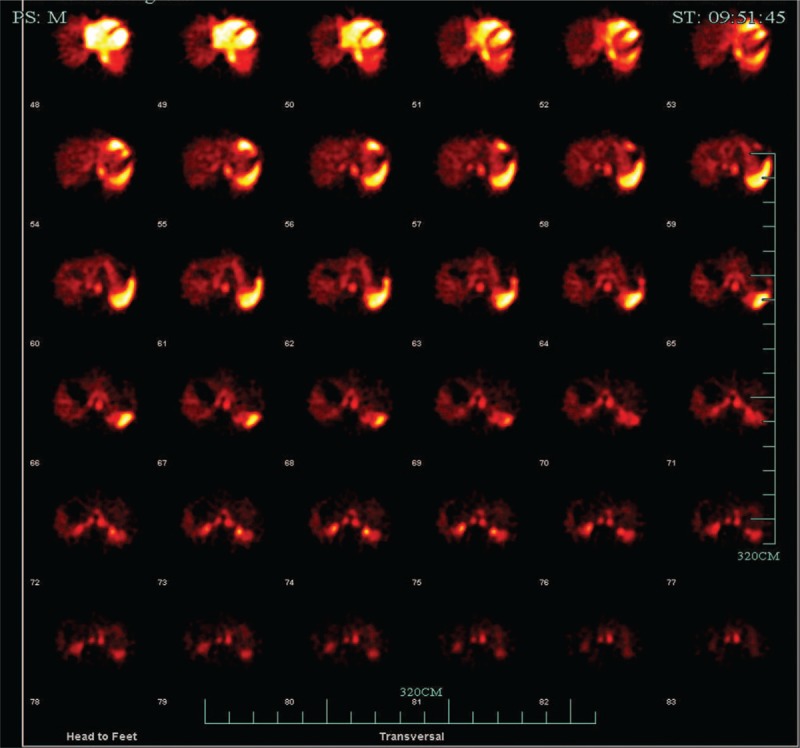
Technetiu-99m–labeled red blood cell scintigraphy.
